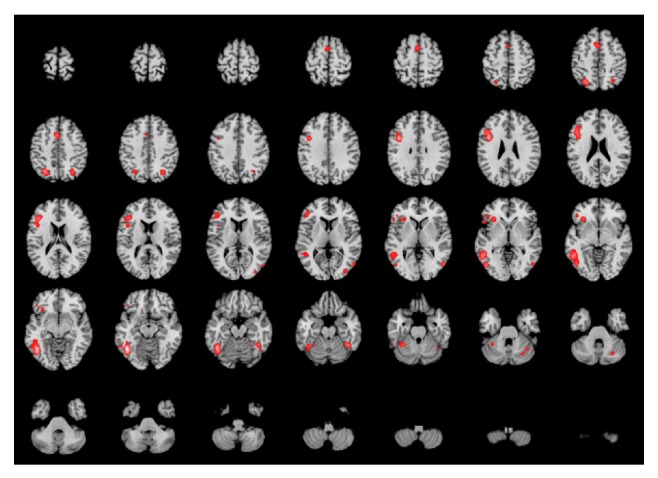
Language-related BA37's network. ALE results overlaid on an axial-T1 MRI MNI-template. Left hemisphere appears in the left side of the insets (neurological convention). ALE scores are color coded from red (lower scores) to white (higher scores). In addition to the left BA37 (middle and inferior temporal gyri in the convexity and fusiform gyrus in the basal aspect) that has the highest intensity, the following regions appear “activated”: left inferior frontal gyrus; bilateral superior and inferior parietal lobule (intraparietal sulcus); left SMA and posterior lobe of the cerebellum. There is also small activation of the right fusiform gyrus and right temporooccipital areas.
